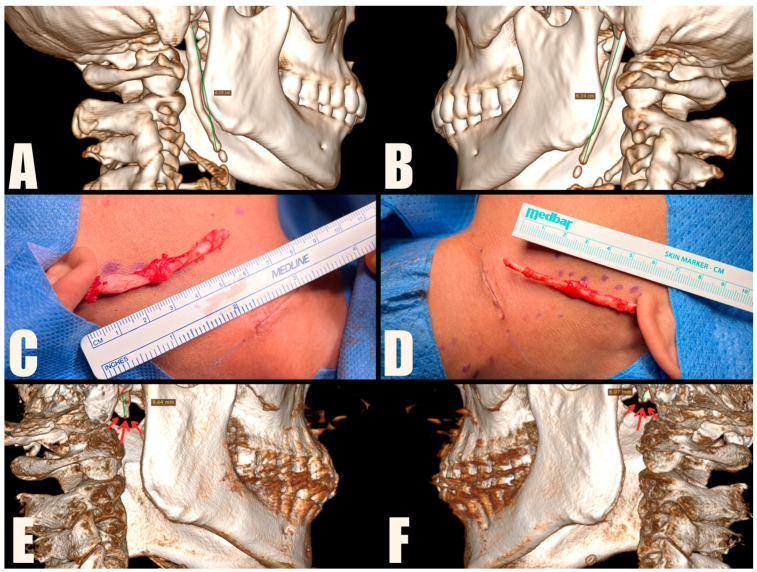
Case presentation of the patient with bilateral ES treated with MICS. (A,B) Preoperative CT scans, with the SP measuring 6.15 cm and the left SP measuring 6.14 cm. (C,D) Intraoperative pictures taken after removing the 6 cm long styloid processes. The wounds are sutured with intradermal sutures. (E,F) Postoperative CBCT scans, indicating residual SP fragments, measuring 9.64 mm on the right and 6.07 mm on the left, highlighted by arrows.
